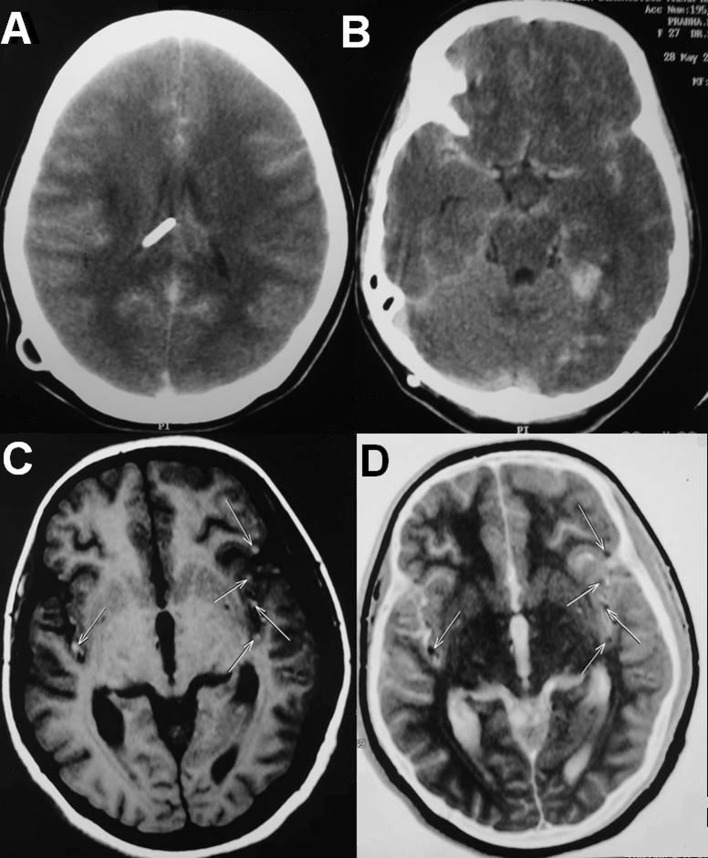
(A, B) Repeat CT scans of the brain showing multiple small tumor emboli with evidence of right temporal bleed and diffuse cerebral edema. (C, D) MRI of the brain showing the tumor emboli on both the hemispheres in middle cerebral artery territory.
